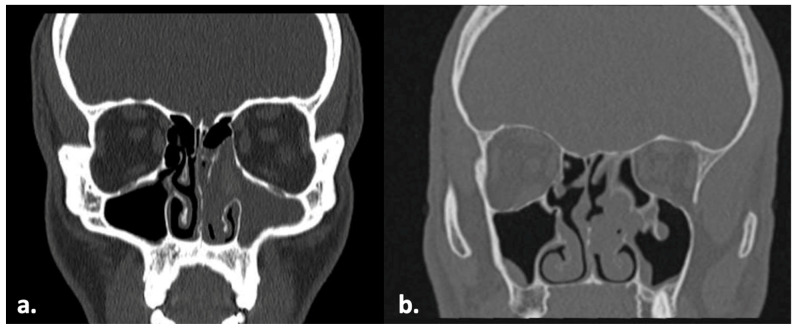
A CT scan of the paranasal sinuses showing the affected maxillary and ethmoid sinus opacification of patient 1 (a) and patient 2 (b) with areas of increased attenuation.
